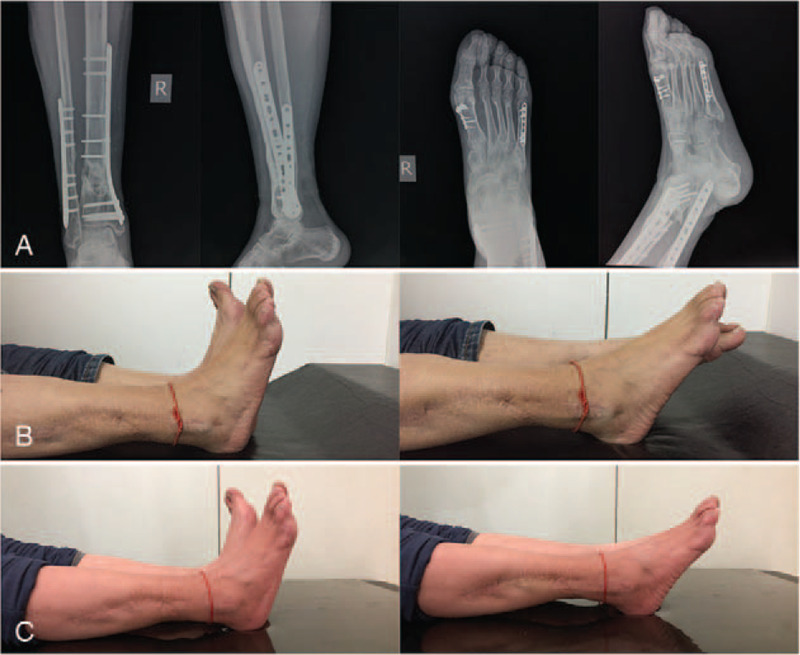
Digital radiograph and motion pictures at 1-year follow-up. Digital radiograph of the lower extremity demonstrates bone union and corrected alignment at the fracture sites on the tibia, fibula, and metatarsals (A). Functional improvement was significant over the first year; however, the flexion and extension of the ankle remained restricted when compared with the contralateral limb (B). At the 2-year follow-up, the function of his right lower extremity was basically essentially fully recovered (C).
